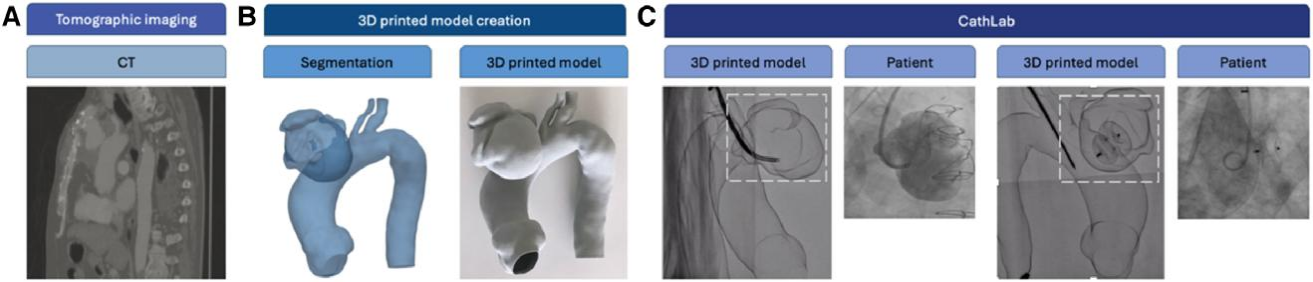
Example of creation and application of a 3D printed model from bench to cath lab: CT image (*A*), 3D printing model creation (*B*), and two different phases of the device deployment in the printed model in the CathLab (*C*).
